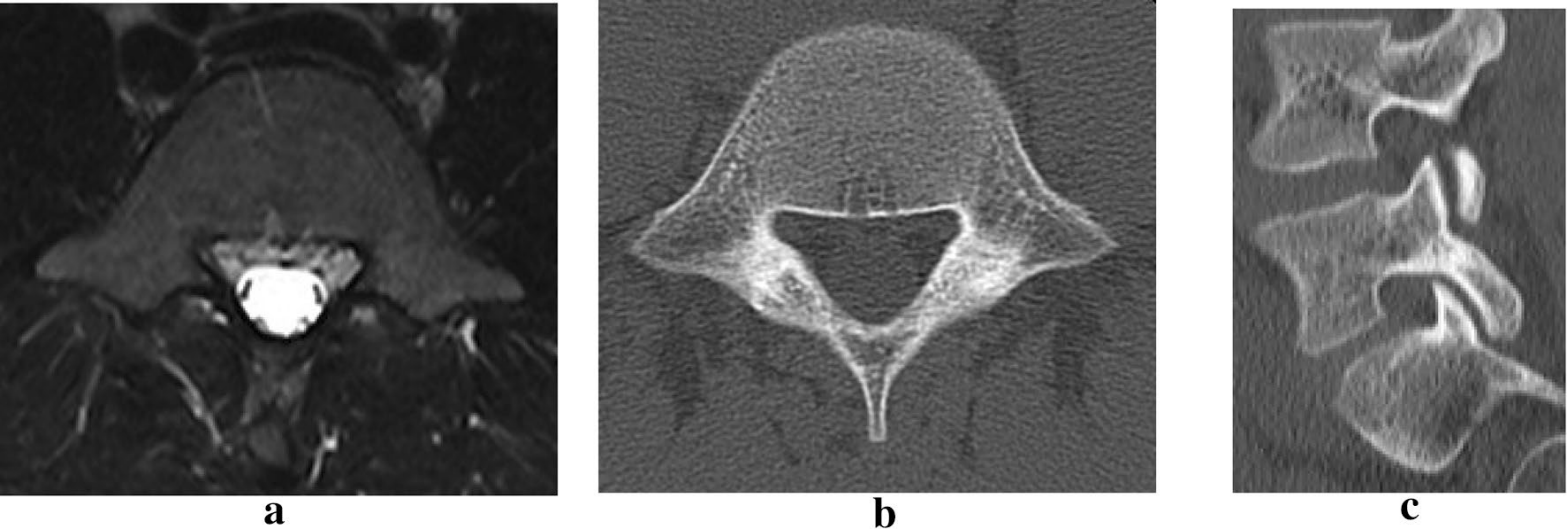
Ten weeks after the second conservative treatment, the intensity change on MRI disappeared (a). No abnormality was detected from the axial or sagittal CT slices (b, c).
